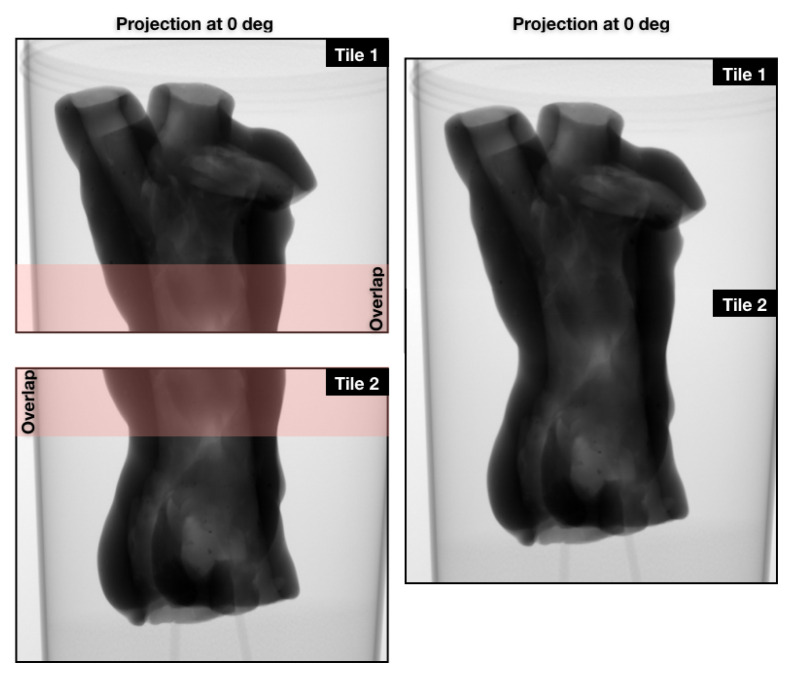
The tile radiographs on the left with the overlapping zone highlighted, and the stitched radiograph on the right.
